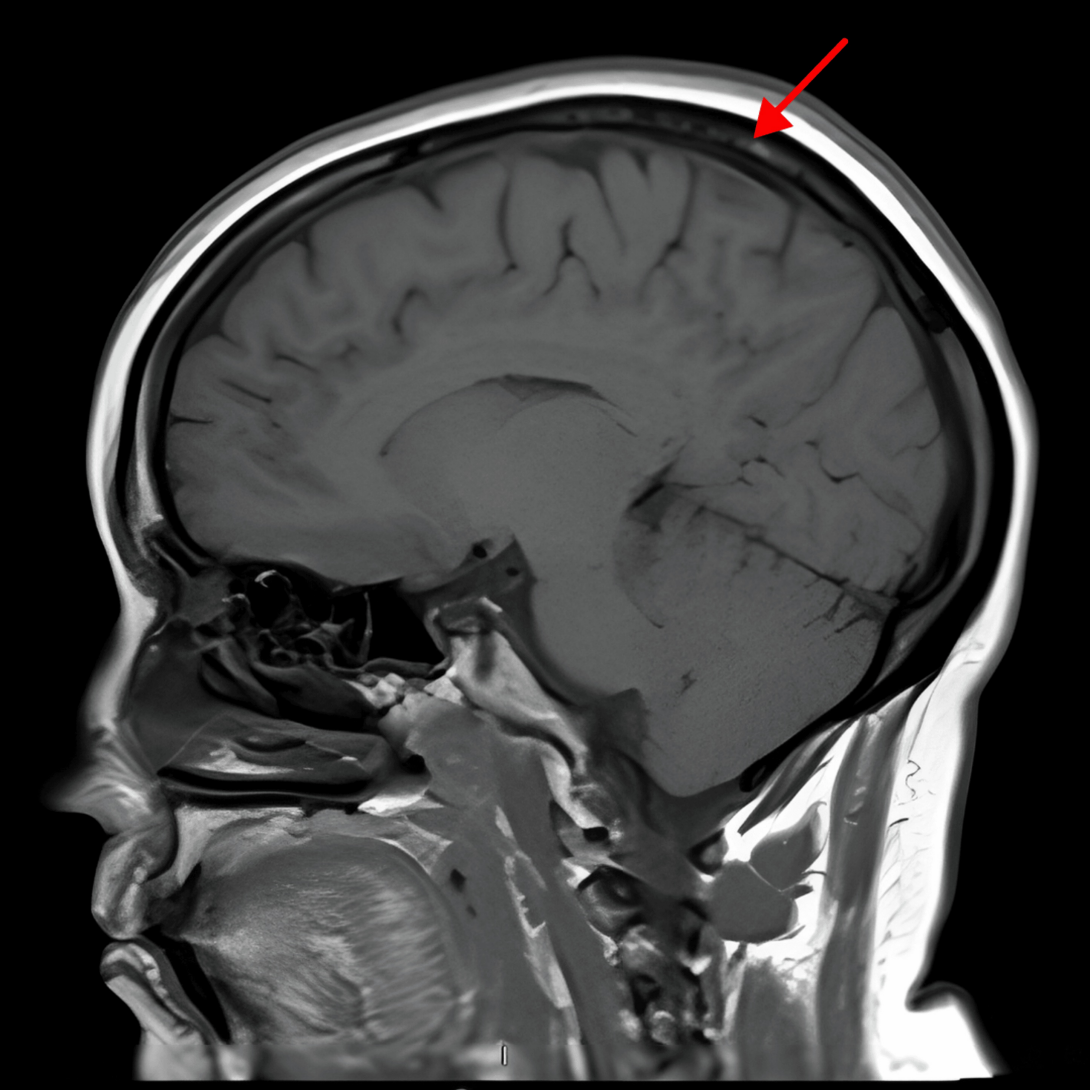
Brain MRI for follow-up post-radiotherapy: right parietal bony lesion shows reduced enhancement with surrounding edema MRI: magnetic resonance imaging
